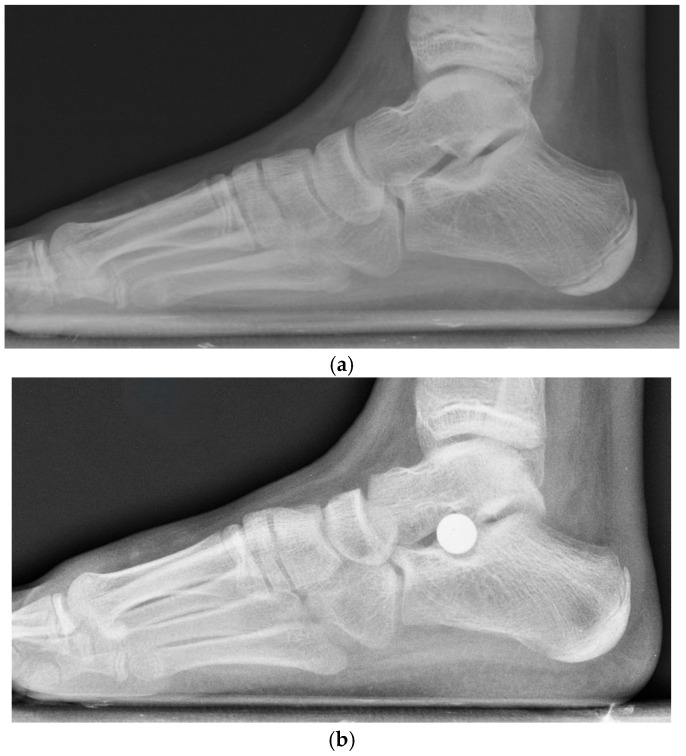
(a) Standing lateral radiograph of the right foot of a 12-year-old girl with a clinical history of recurrent ankle sprain and occasional pain on the lateral aspect of the ankle. She had a misdiagnosis of flatfoot because Meary’s angle measured −4°, while the other angles indicating flatfoot were normal. (b) An endosinotarsal implant was applied, with overcorrection of the misdiagnosed FF. As a result, ankle sprains became more frequent, and ankle pain worsened.
